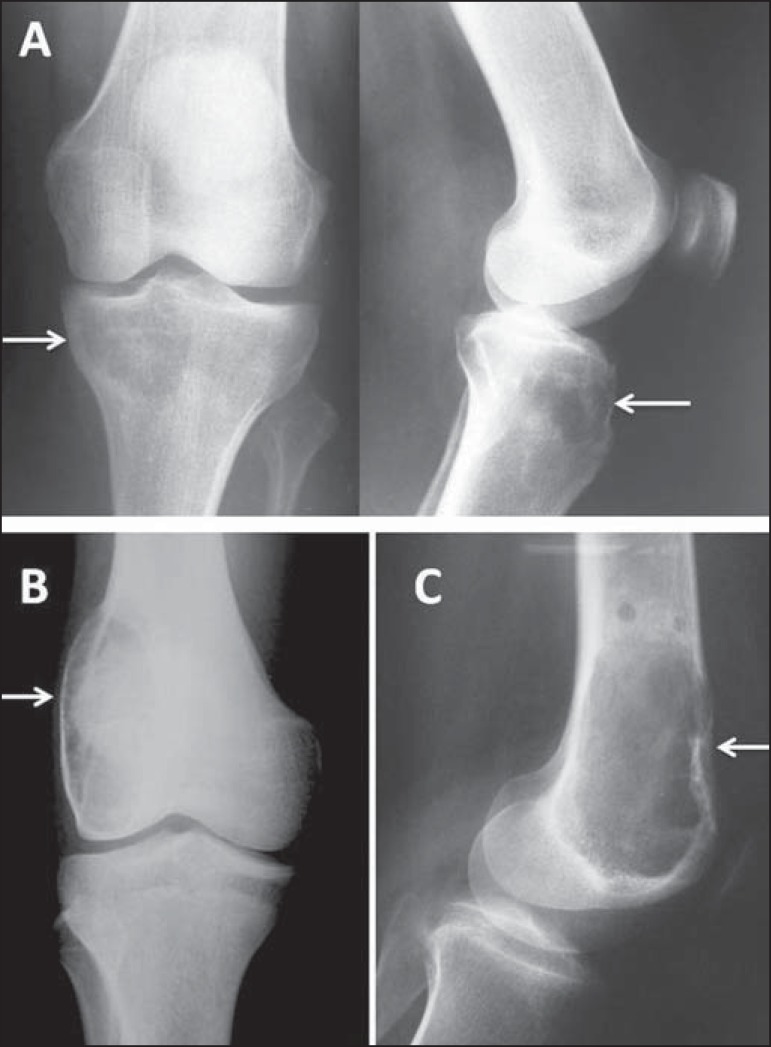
A: Adult female patient with recent knee pain; radiography
